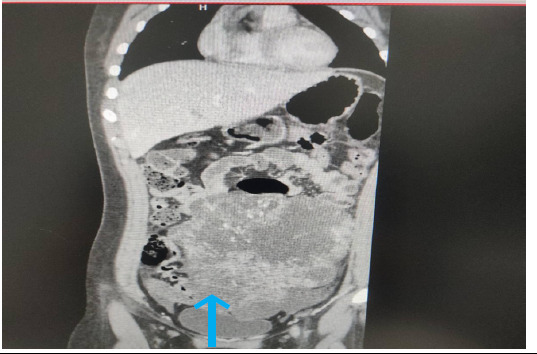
CT abdomen pelvis showing yolk sac tumour.
